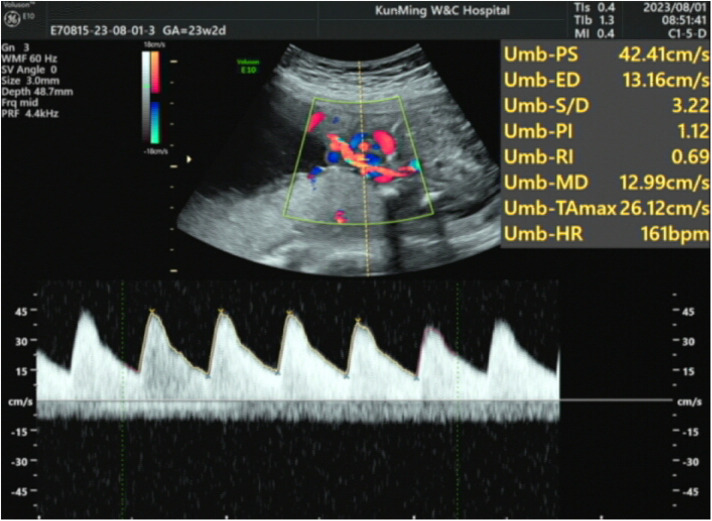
The patient underwent a fetal systemic ultrasound examination at 23 weeks and 2 days of gestation, which revealed a VSD; enlargement of the right atrium and right ventricle with mild tricuspid valve regurgitation; and narrowing of the left and right pulmonary arteries.
